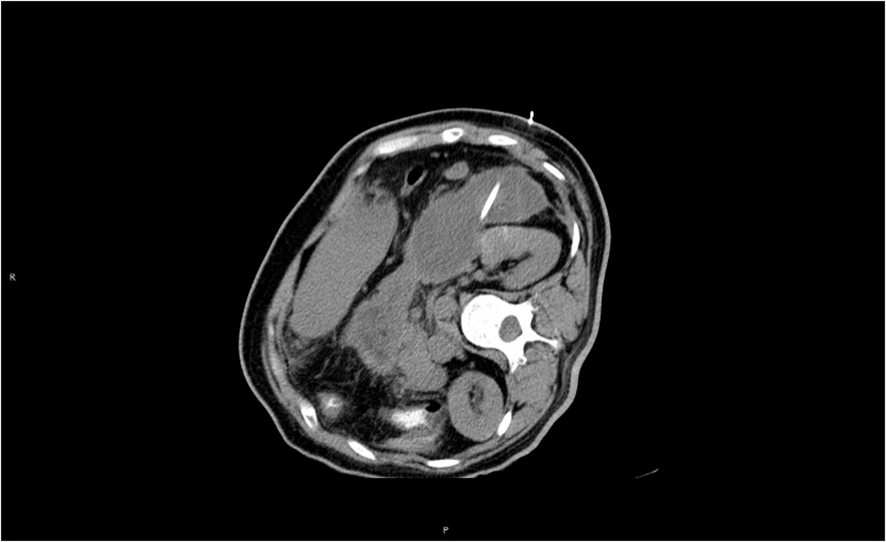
Peripancreatic fluid with infection, CT-guided puncture, and drainage was performed.
